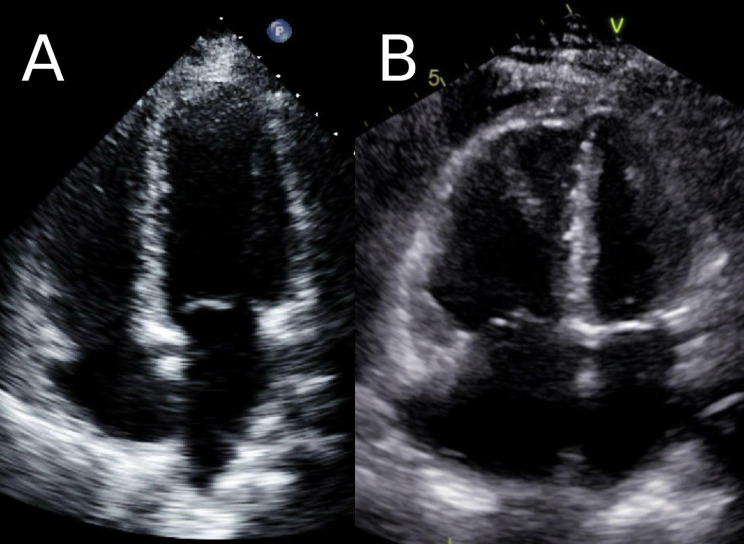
Representative echocardiographic images from patients with systemic sclerosis-associated heart failure with preserved ejection fraction (SSc-HFpEF). (A) Isolated postcapillary pulmonary hypertension (Ipc-PH) phenotype. (B) Combined pre- and post-capillary pulmonary hypertension (Cpc-PH) phenotype, showing larger right atrial and right ventricular chamber dimensions
